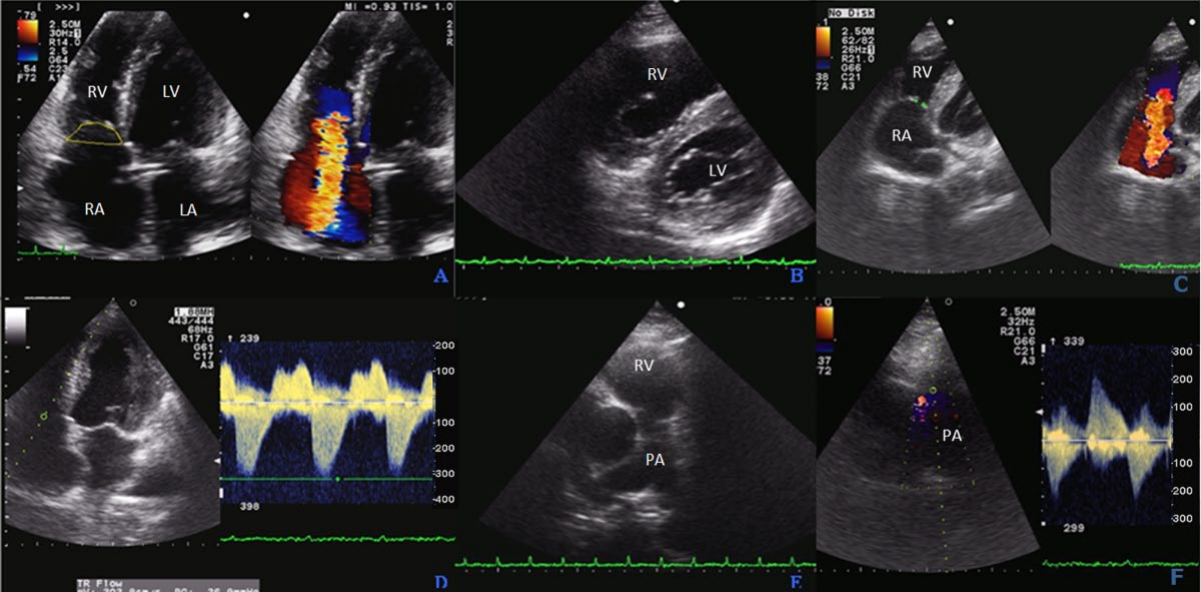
Echocardiographic findings: (A) Four-chamber view showing severe tricuspid regurgitation into a dilated right atrium. On the left, tenting area. (B) Short-axis view showing “D-shaped” left ventricular morphology as sign of right ventricular overload. (C) Tricuspid valve detail showing thickened and retracted valve leaflets failing to coapt and resulting in constant “semi-open” position. (D) Continuous wave (CW) Doppler of the tricuspid valve confirming severe regurgitation. (E) Pulmonary valve with thickening of pulmonary cusps and dilated pulmonary trunk. (F) CW Doppler of the pulmonary valve showing moderate valve stenosis and severe regurgitation with pulmonary hypertension (acceleration time 80 ms). RA, right atrium; RV, right ventricle; LA, left atrium; LV, left ventricle; PA, pulmonary artery.
